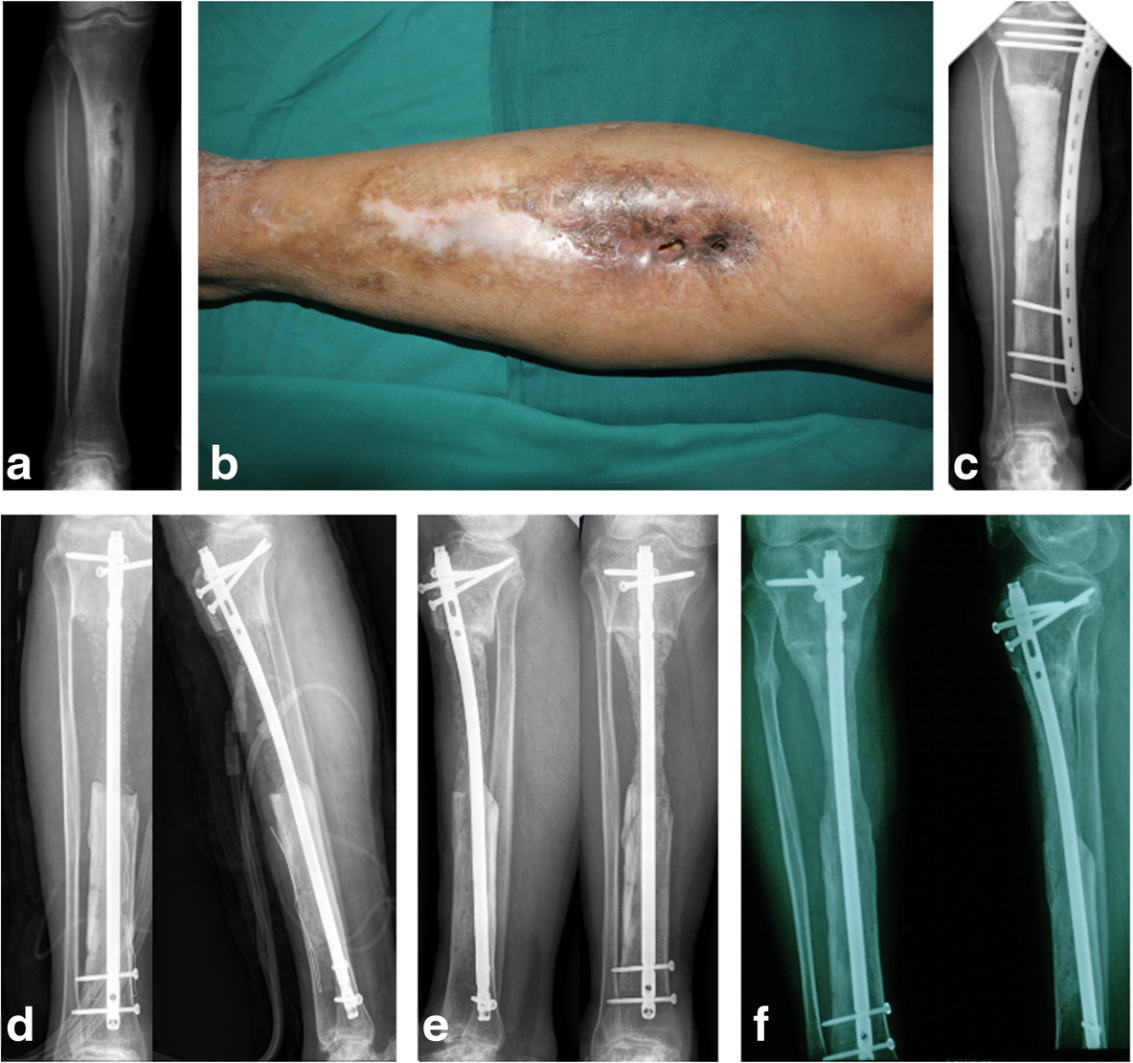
Case 10, 50 Y, repeated swelling and skin ulcer of the right proximal tibia for more than 30 years. *Proteus mirabilis* was identified. a and b: Sinuses were observed, and X-ray revealed bone destruction; c: Complete resection of the lesions and implantation with PMMA cement after the second stage; d: Grafting (autograft 60 ml + allograft 15 ml) eight weeks later; e: X-ray revealed bone union at 6 months; f: 20 months after the second stage, bone union with a small diameter was noted
